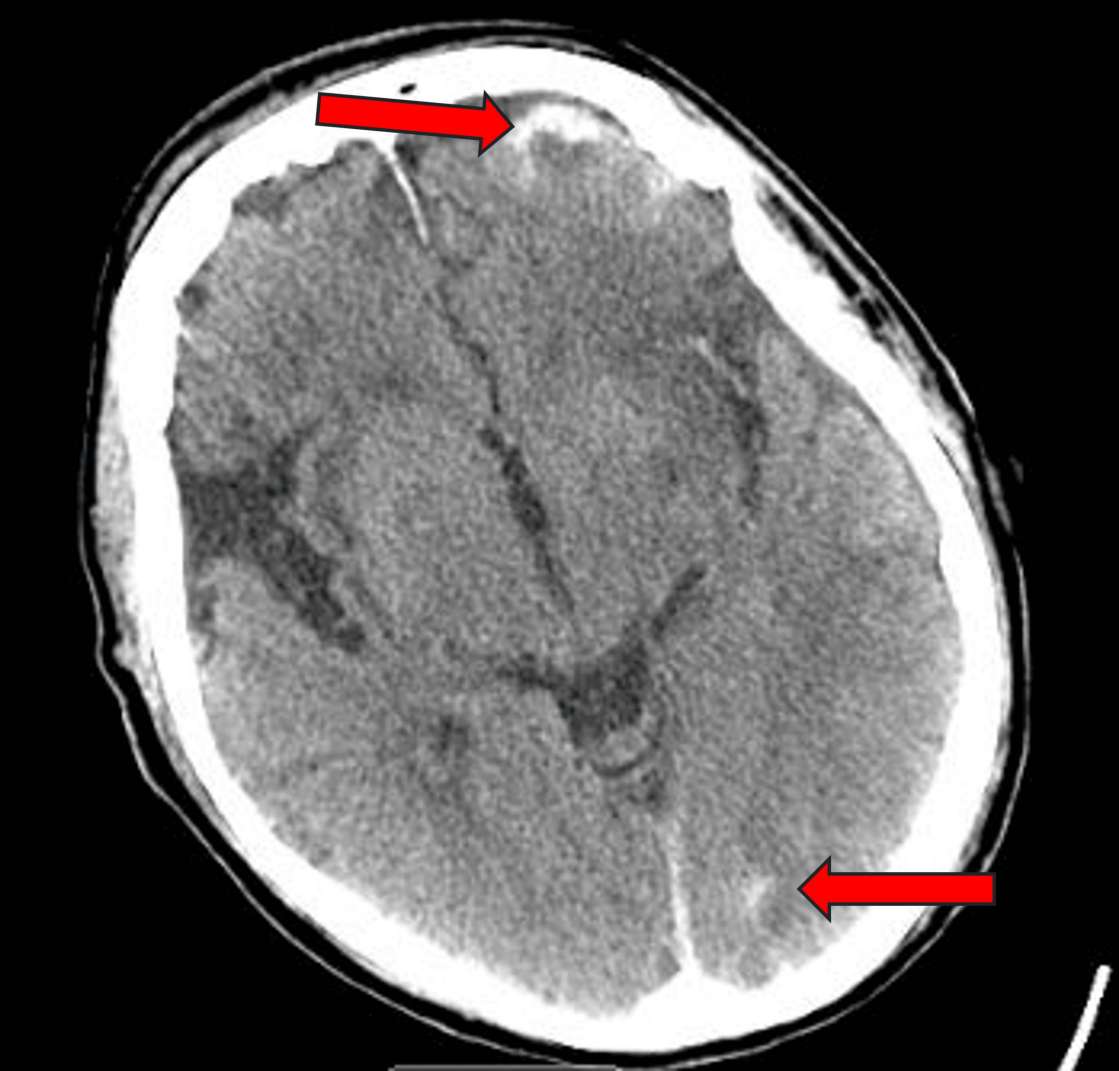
Patient 2 head CT without contrast Subarachnoid hemorrhage to the left frontal lobe, left parietal lobe, and subdural hemorrhage to the left frontal region (top arrow), and a left occipital lobe hemorrhage (bottom arrow).
